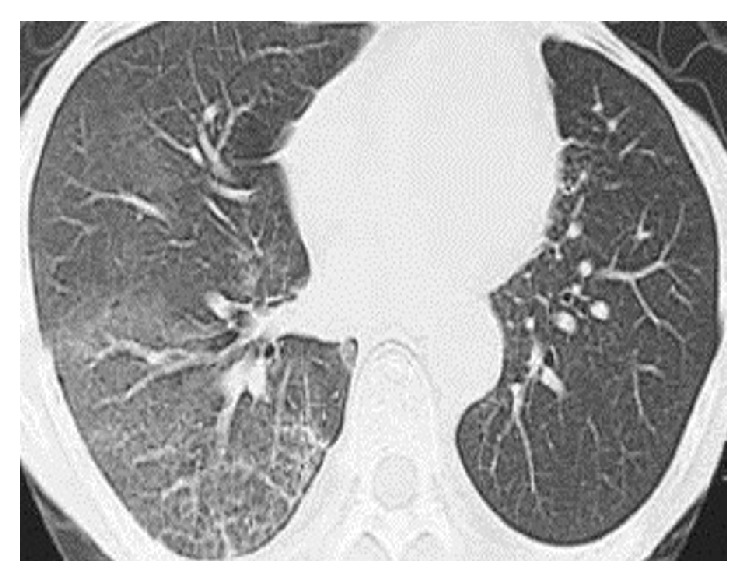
A section of HRCT done prior to discharge showing dramatic improvement in the lung filed shadowing.
